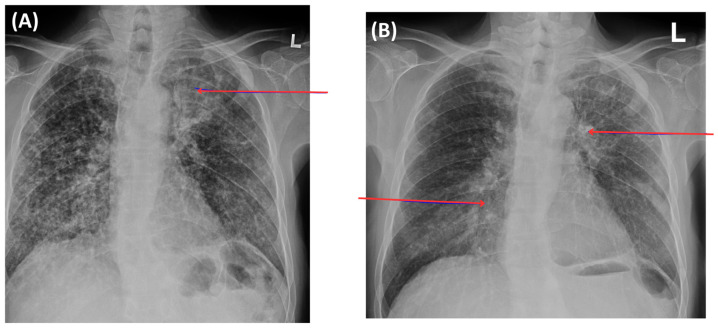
Chest X-ray (15 August 2024) at base line compared to the most recent: left upper lobe mass opacity with diffuse interstitial thickening (A); follow-up imaging (5 September 2025) revealed left lung opacity showing treatment response [red arrow left] and there is redemonstration and improvement in diffuse interstitial thickening [red arrow right] (B).
